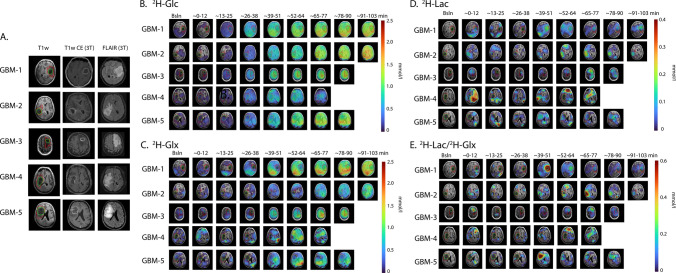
A MR images displaying a transversal slice at the location of the tumor. The tumor (red) and tumor core (green) ROI are drawn on the T_1_-weighted image (T_1_w) acquired at 7T. The T_1_-weigthed contrast-enhanced images (T_1_w CE) and the fluid-attenuated inversion recovery (FLAIR) images were acquired at 3T in the weeks prior to the DMI scan. (B-E) Dynamic DMI maps in the same transversal slice of ^2^H-Glc B, ^2^H-Glx C, ^2^H-Lac D and ^2^H-Lac/^2^H-Glx E after oral consumption of [6,6’-^2^H_2_]glucose
